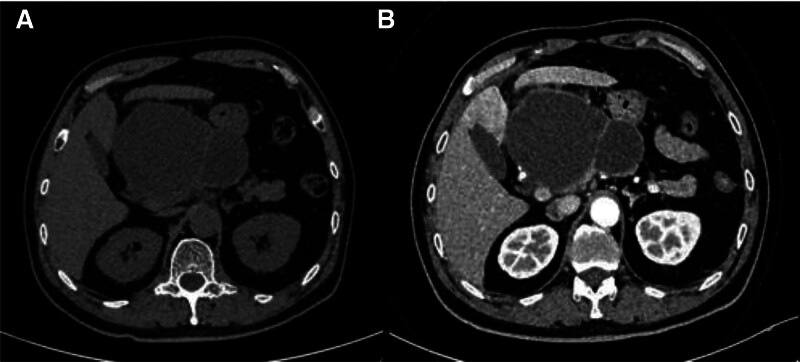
Noncontrast (A) and arterial phase CT (B) scans showing a cystic mass in the head and body of the pancreas. The density of the cystic fluid is slightly higher than that of water, with a few patchy areas of slightly higher density floating within. The cyst wall and septa show mild enhancement, while the contents do not enhance. CT = computed tomography.
